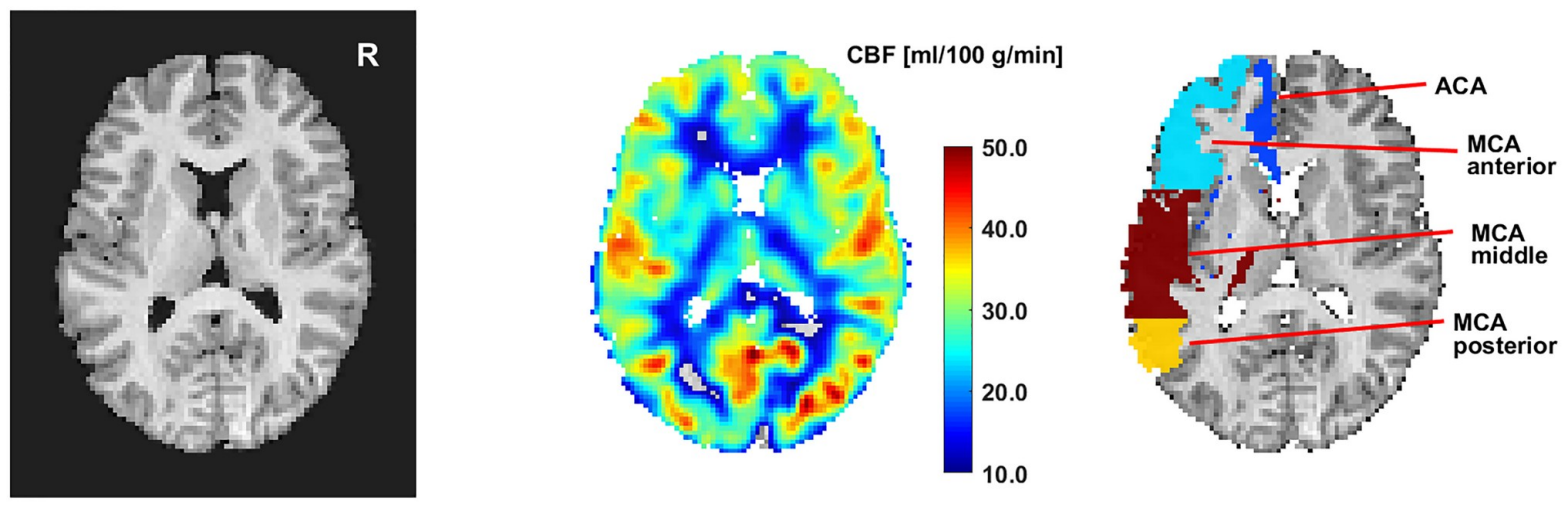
MRI, ASL perfusion map and analyzed brain regions. The left panel shows an axial brain MRI, the middle panel an ASL perfusion map with colors indicating the regional cerebral perfusion in ml/100g/min. The right panel shows the analyzed vascular territories of the anterior cerebral artery (ACA), middle cerebral artery (MCA) and subregions (MCA anterior, middle, posterior).
